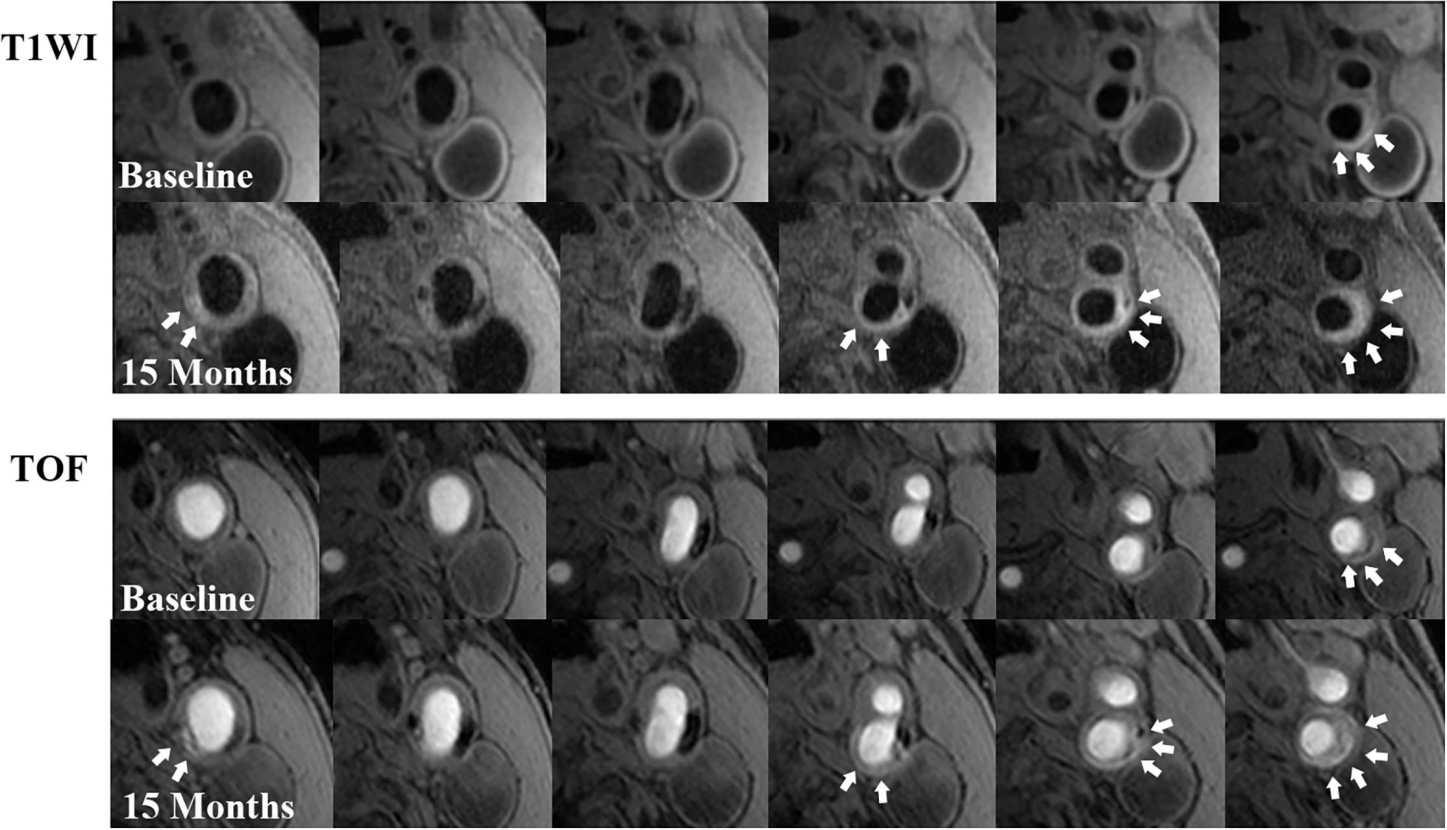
Examples of IPH progression during a 15-month follow-up. There is a remarkable progression in IPH volume (white arrows) at left carotid bifurcation with atherosclerosis on T1W (above 2 rows) and TOF (below 2 rows) images from the baseline to the follow-up. In addition, new IPHs (white arrows) could be observed at other locations of the plaque on follow-up MR images compared with those at baseline.
